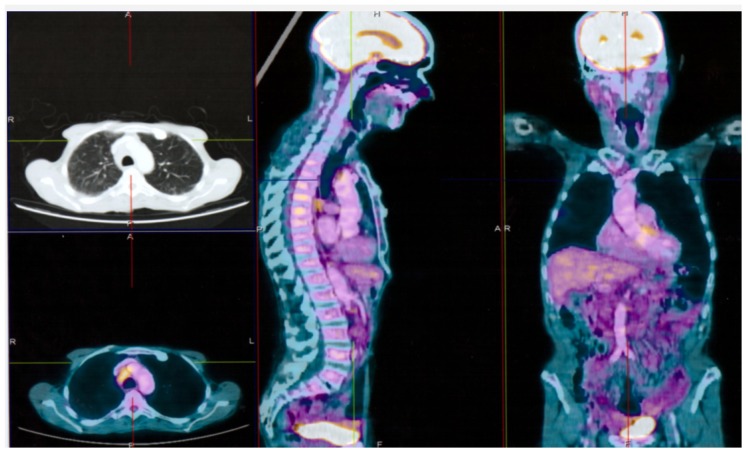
[18F] FDG-PET performed with co-registered CT in follow up (October 2019) not show significant areas of increased metabolic activity in abdominal cavity.
